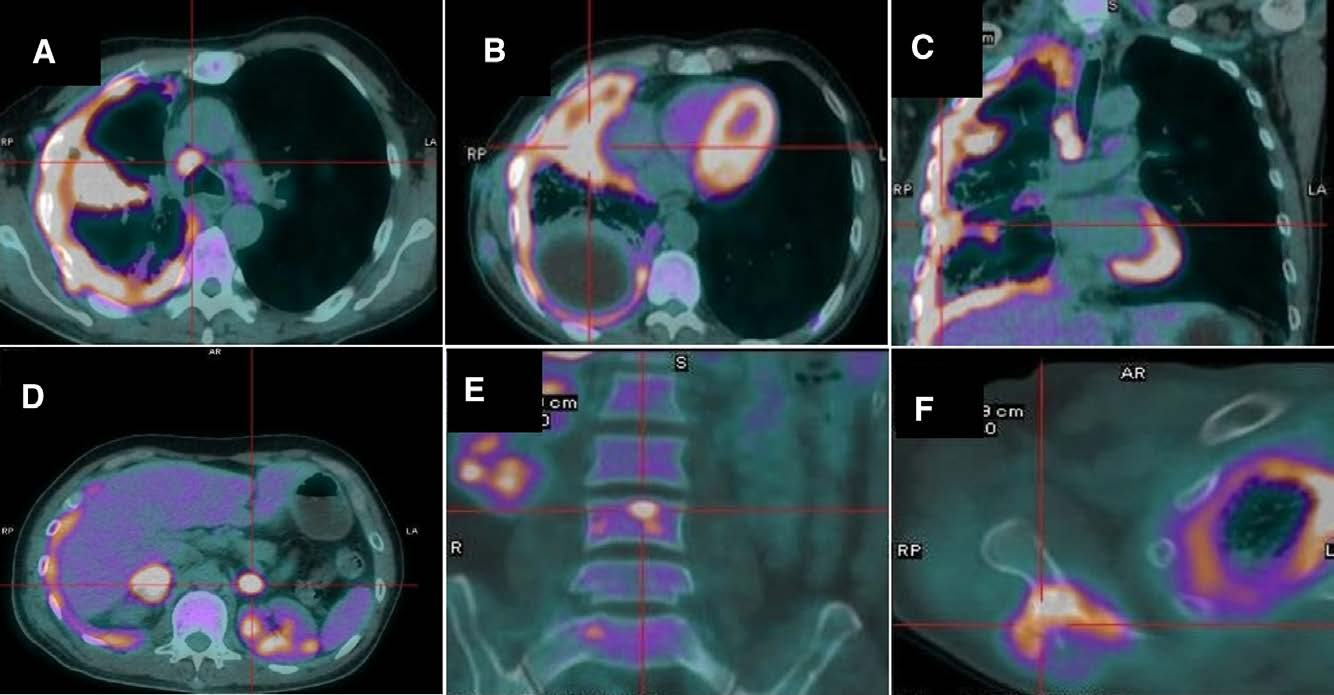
PET-CT scanning. PET-CT showing an abnormal increase in glucose metabolism of the right lung mass, enlarged mediastinal lymph nodes, and diffuse thickening of right pleura. In addition, systemic metastases in bilateral adrenal glands, right scapula, and multiple vertebrae were revealed.
